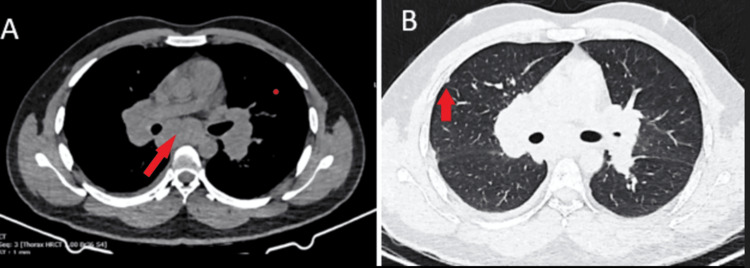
(A-B) High resolution CT chest – mediastinal lymphadenopathy with subpleural nodules The arrow in 1A points to subcarinal LN; the arrow in 1B points to subpleural nodule. LN: Lymph node
